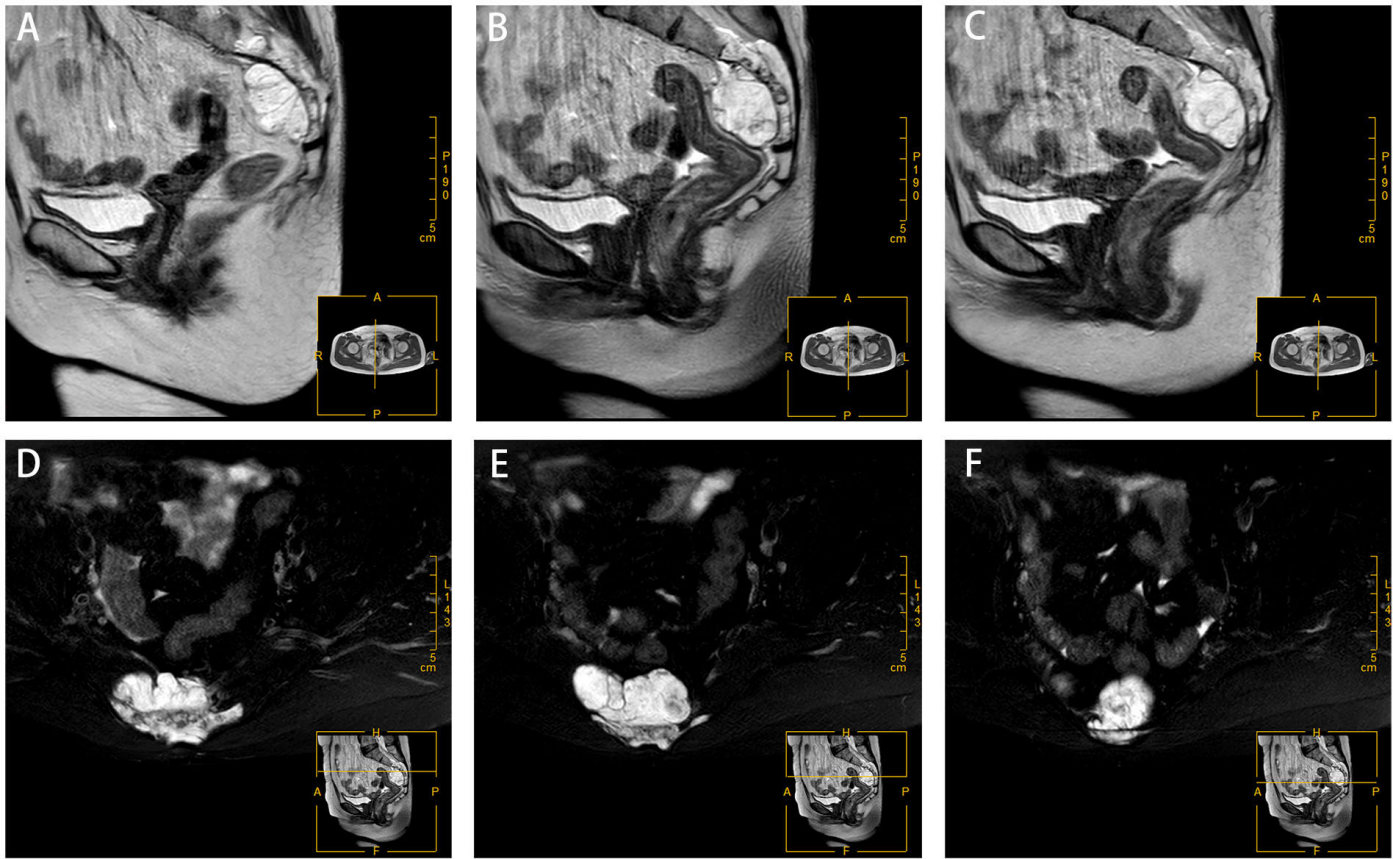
Magnetic resonance imaging at the time of diagnosis. Clumpy long T1 (A-C) and long T2 (C-E) signals are seen in and around the S2-5 vertebrae.
